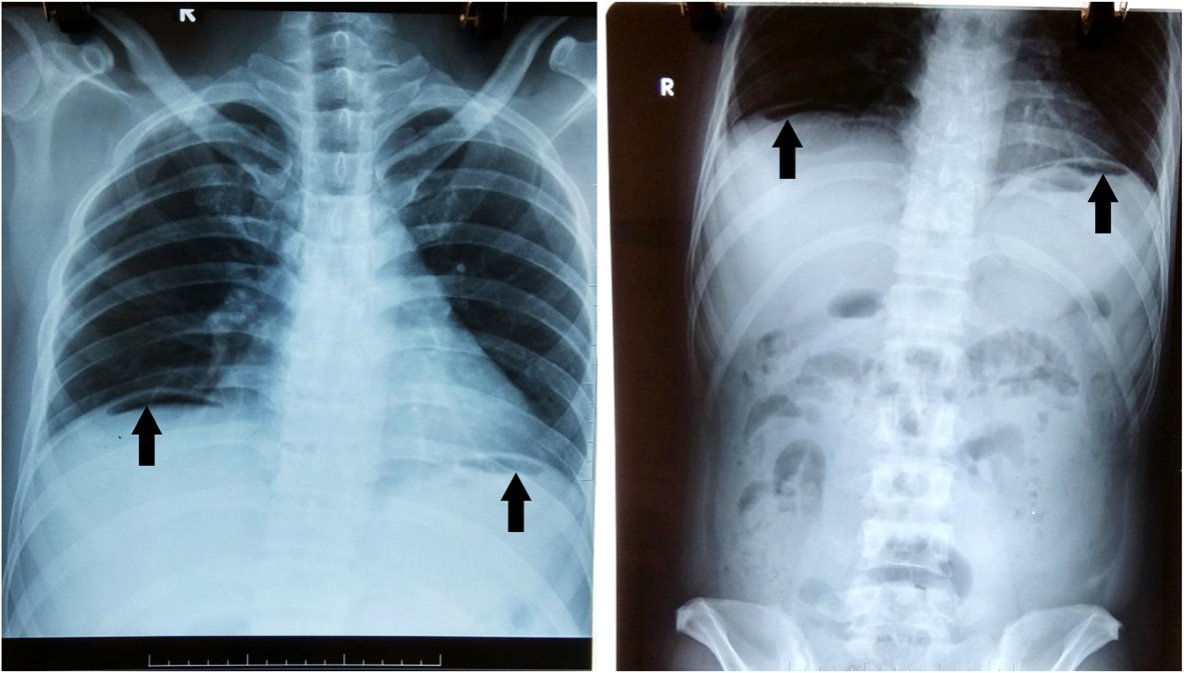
Gas under both domes of diaphragm on radiograph. Routine preoperative X-ray taken on admission showed free gas under both domes of diaphragm (arrows)
